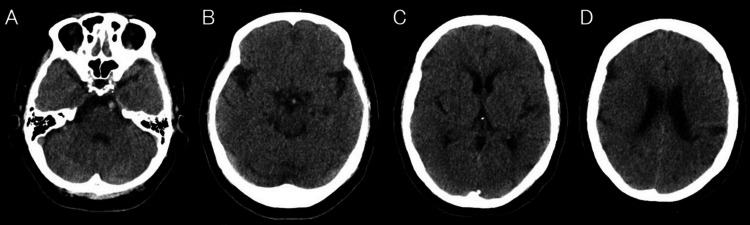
(A-D) Initial noncontrast-enhanced head CT scan performed three hours after symptom onset reveals no hemorrhage or early ischemic changes. (A,B) The basilar artery appears hyperdense CT: computed tomography
